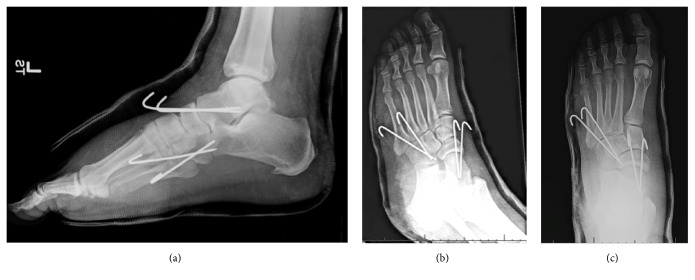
Postoperative radiographs demonstrating stabilization of the fourth and fifth tarsometatarsal joints and talonavicular joints with pins.
